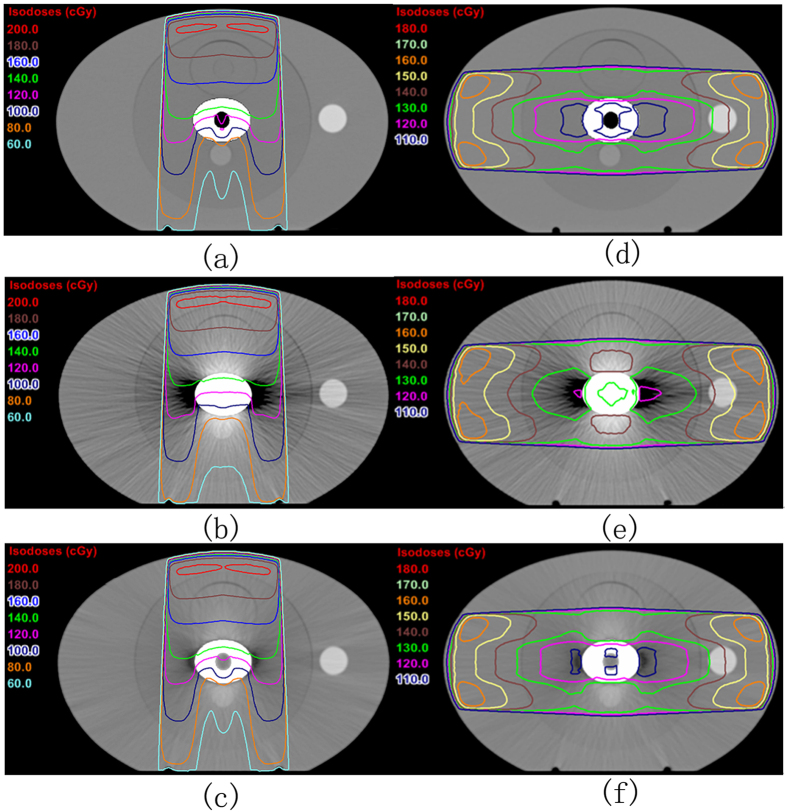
Dose distribution of different CT images in single front-field irradiation plan (a), (b), (c) and horizontal opposed field (d), (e), (f) designed by TPS. (a) and (d) are theoretical CT images, (b) and (e) are uncorrected kVCT images, (c) and (f) are corrected images.
